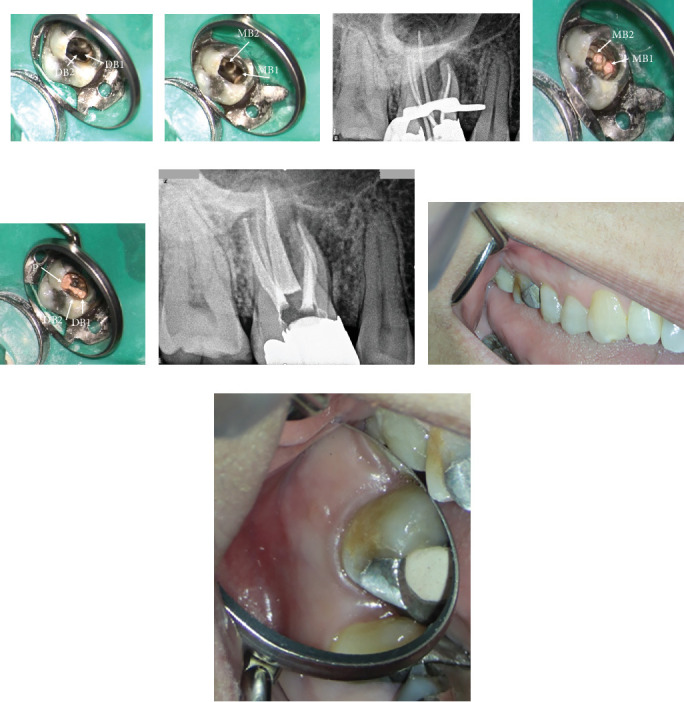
(a) Identification of missed DB2 canal. (b) Identification of missed MB2 canal. (c) Master cone fitness of all five canals. (d) The orifices of obturated MB1 and MB2 canals in the pulp floor. (e) The orifices of obturated DB1 and DB2 canals in the pulp floor. (f) Radiographic view of final obturation in five canals. (g, h) Clinical image showing resolution of the buccal swelling.
